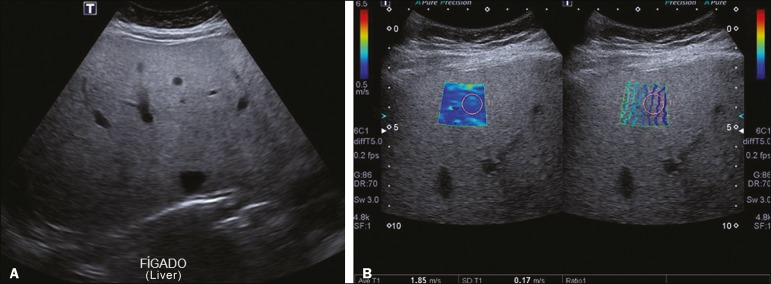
A: Ultrasound examination of a liver with marked steatosis, as characterized by increased echogenicity of the liver parenchyma and posterior attenuation of the ultrasound beam. Attenuation of the ultrasound beam obscures the view of the diaphragm. B: A 2D-SWE study showing greater stiffness of the liver parenchyma (1.85 m/s), consistent with a Metavir stage of F3. The blue and green tones are in equal proportions on the elastography map and the lines are further apart in the propagation mode.
